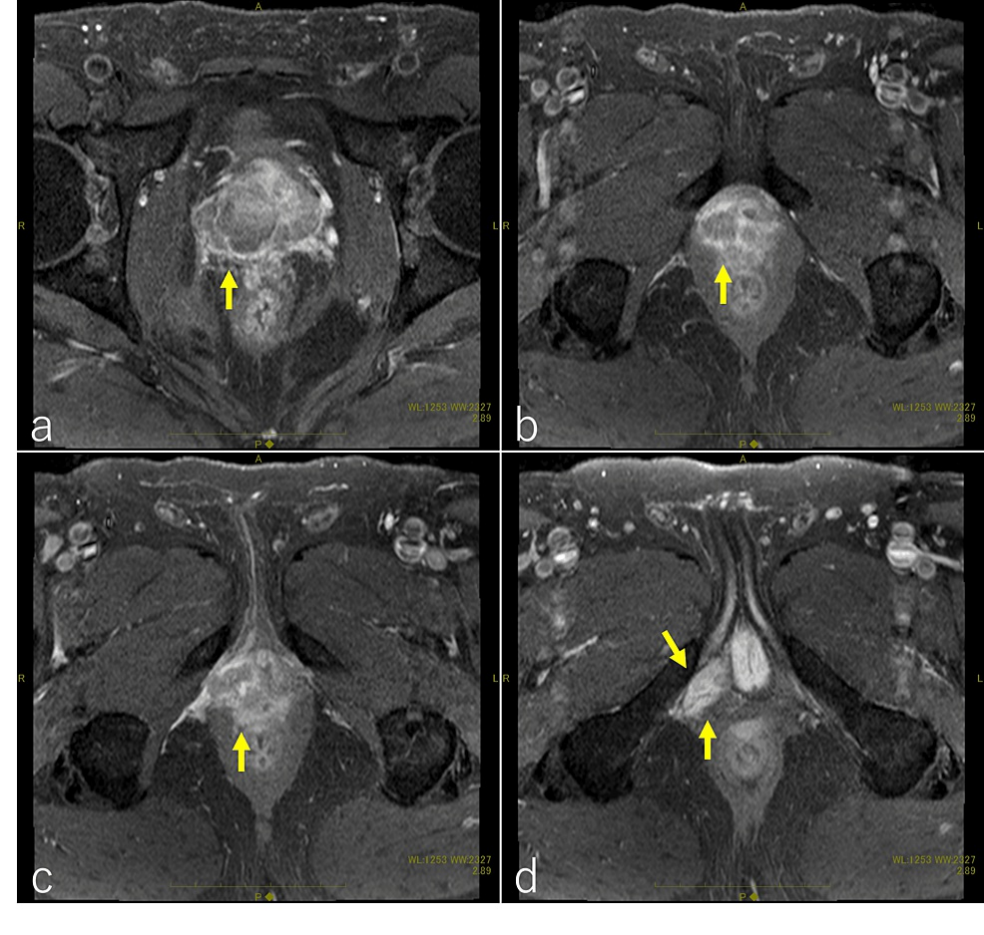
Pelvic contrast-enhanced MRI The MRI reveals a mass lesion in the prostate, consistent with the CT findings. The prostate is primarily enlarged on the right side, and a contrast-enhancing mass is visible (indicated by arrows in a and b). There is infiltration toward the apex of the prostate (indicated by an arrow in c), with suspected involvement of the pubococcygeal and puboanal muscles, along with infiltration of the intraosseous trabecularis muscle and the superficial transverse perineal muscle (indicated by arrows in d).
